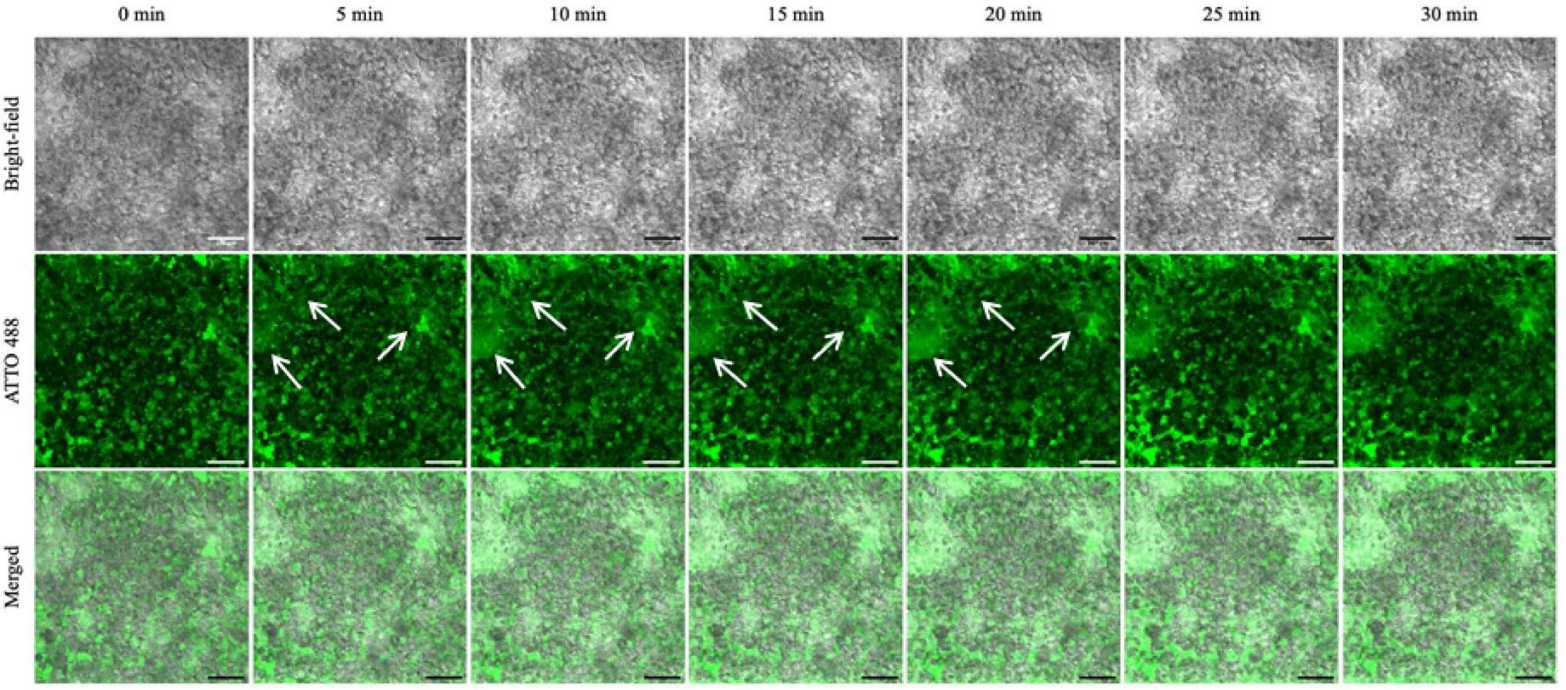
Confocal images of the ATTO 488 fluorescence intensity in the 21-day microfluidic PDAC culture with repeated ultrasound exposure. Gemcitabine and microbubble solution was perfused on-chip with the PDAC culture for 2 h to allow bubbles in the media channel to penetrate the PDAC culture. With microbubbles in the culture chamber, ultrasound was applied every 5 min for 30 min. White arrows indicate the changes in the ATTO 488 signal from the microbubble shell during the 30-min US exposure. Scale bar, 100 µm.
